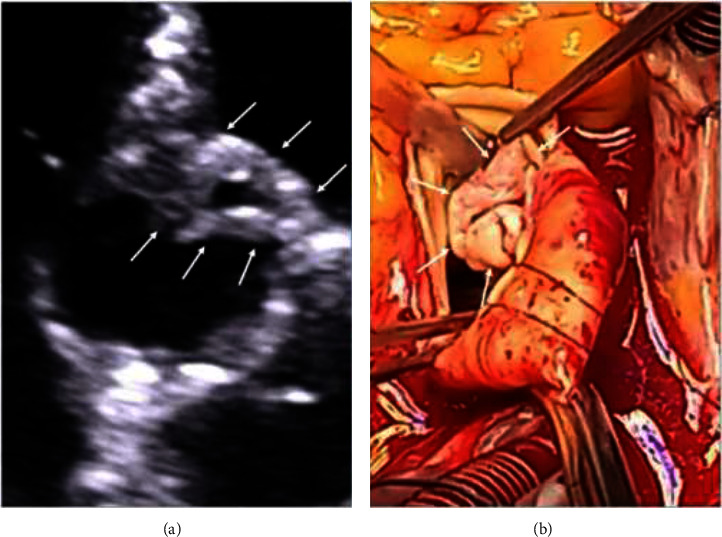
Large aortic valve vegetation found during surgery (a) and image comparison with echocardiographic presentation (b). The white arrows indicate vegetation.
